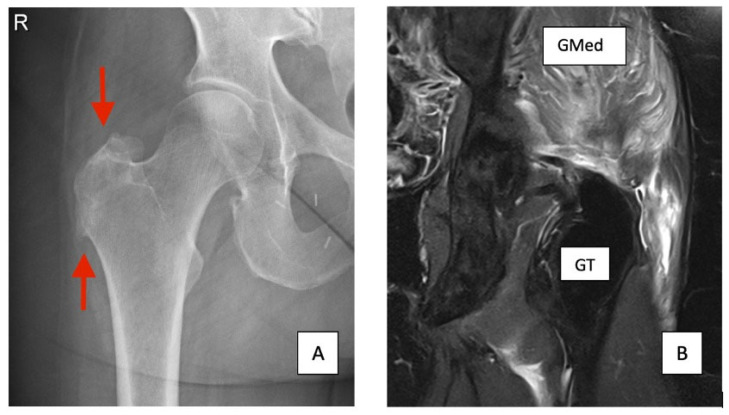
(A) Standing AP Xray of the Right Hip with distal spur of the GT and irregular cortex (red arrows) (B) T2 MRI Left hip with fatty degeneration of the GMed and liquid filled bursa and discontinued Gluteal tendon.
